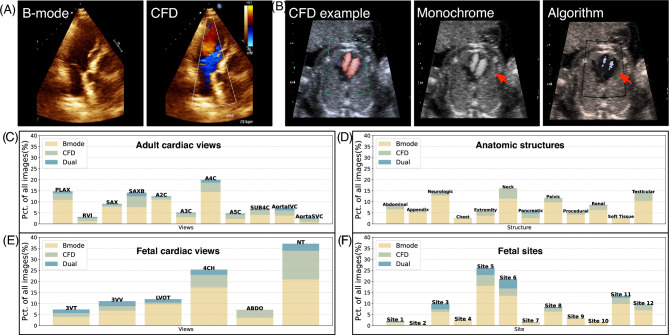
B-mode and color flow doppler (CFD) offer different information, belie simple translation methods, and are unbalanced in datasets. (A) Example B-mode and corresponding CFD ultrasound. (B) Monochrome rendering makes the CFD signal grey (red arrow) instead of matching the blood pool. Algorithmically detecting colored pixels and replacing them with grayscale values has several problems, including retaining the CFD region of interest box, imperfect thresholds for colored pixels, and replacement greyscale values that do not match the color or noise profile of the ultrasound image at hand (red arrow). Furthermore, anatomic structures underneath the original color signal are not reconstructed. The prevalence of B-mode, CFD, and dual acquisitions is imbalanced and varies across typical clinical views (C, E); different anatomic structures (D); and even across different clinical sites (F). *PLAX* parasternal long axis, *RVI* right ventricular inflow, *SAX* short axis mid or mitral, *SAXB* basal short axis, *A2C* apical two chamber, *A3C* apical three chamber/long axis, *A4C/4CH* four chamber, *A5C* apical five chamber, *SUB4C* subcostal four-chamber, *AortaIVC* abdominal aorta or subcostal inferior vena cava, *AortaSVC* aortic arch or superior vena cava, *3VT* three-vessel trachea, *3VV* three-vessel view, *LVOT* left-ventricular outflow tract, *ABDO* abdomen, *NT* non-target.
